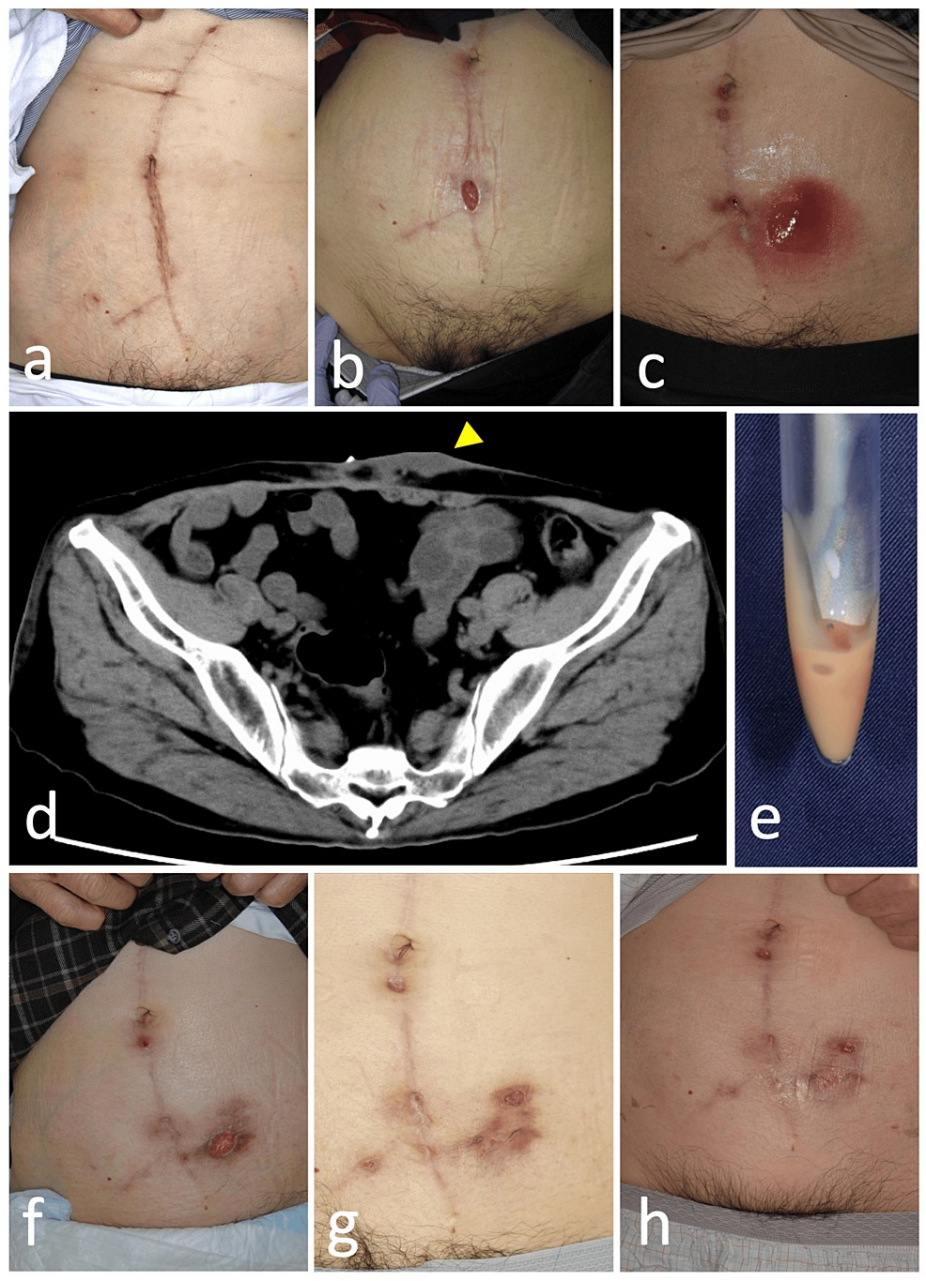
Recurrent mobilization of abdominal wall infection (continuation of case 1). No recurrence of infection was observed three weeks after mesh removal (a). Two months post-mesh removal, a skin ulcer with erythema developed (b). Despite local treatment with topical medications and oral antibiotics, a new, severely erythematous abscess formed four months after mesh removal (c). On the same day, a CT scan revealed a subcutaneous abscess (arrow head); aspiration yielded purulent fluid (e). Six months (f), eight months (g), and nine months (h) post-mesh removal, the ulcerated areas progressively expanded in a stepping-stone pattern.
